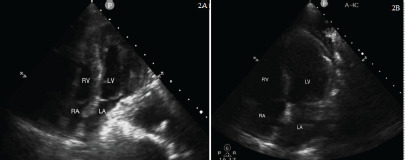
(2A) Apical 4-chamber cardiac view from point-of-care ultrasound of the left atrium immediately after nasogastric tube (NG) suction of approximately 600 milliliters of content. (2B) The same view of the left atrium after continued NG tube suctioning the following morning. *RA*, right atrium; *RV*, right ventricle; *LV*, left ventricle; *LA*, left atrium.
